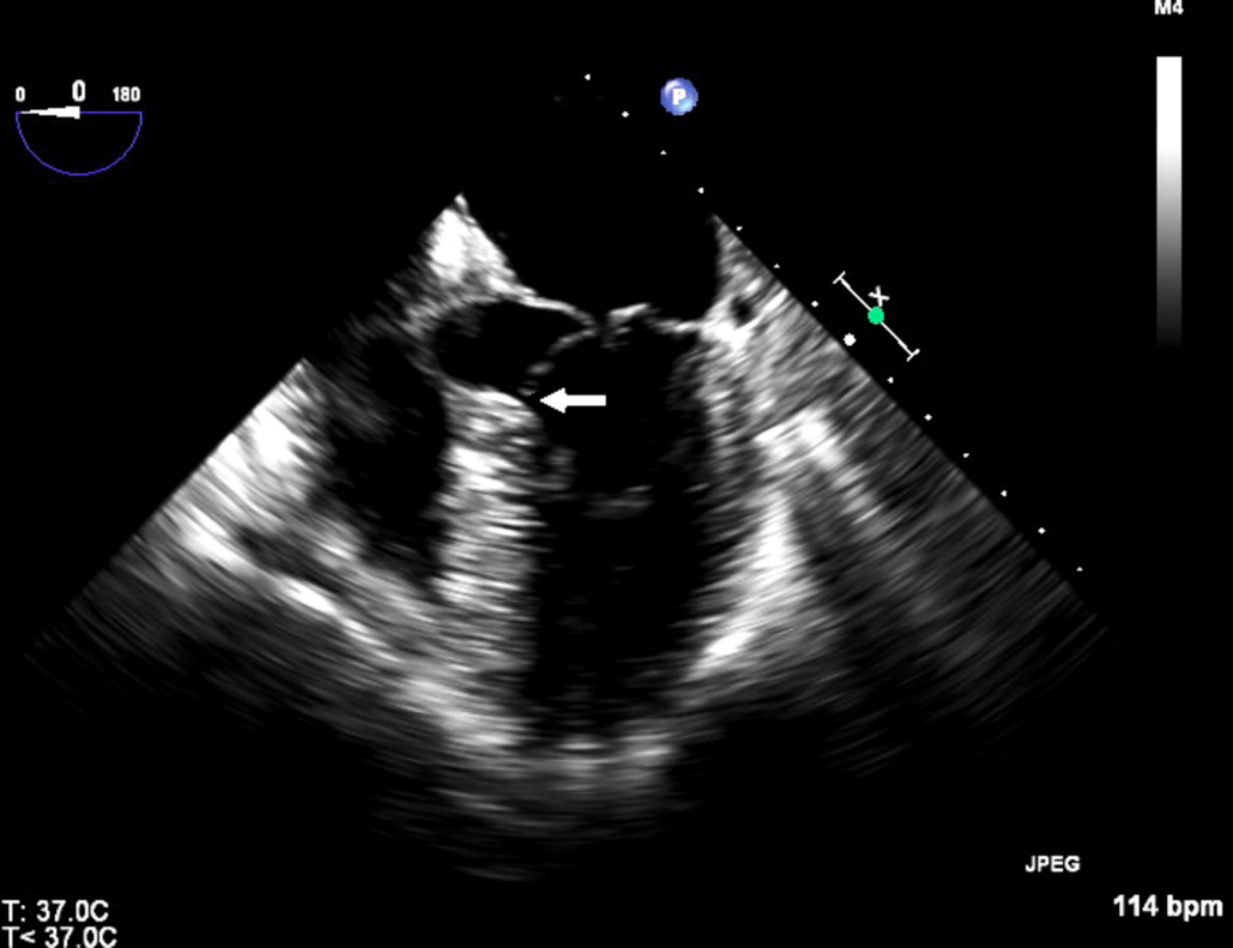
Transesophageal echocardiographic image showing systolic anterior motion of the anterior mitral valve leaflet (white arrow)
